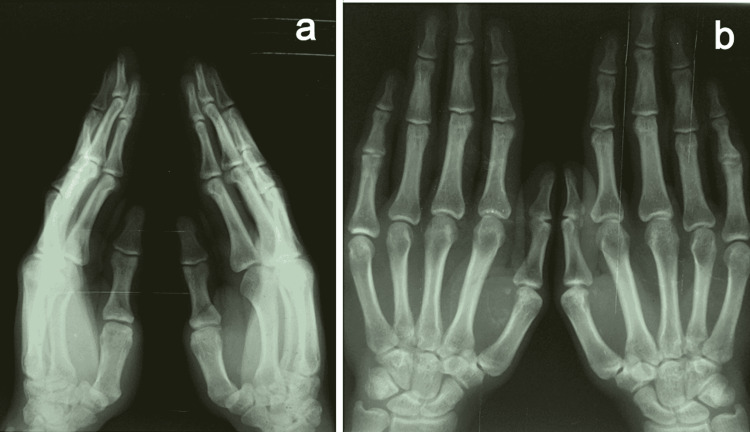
X-ray of the hand. (a) Lateral view. (b) Anteroposterior view.
